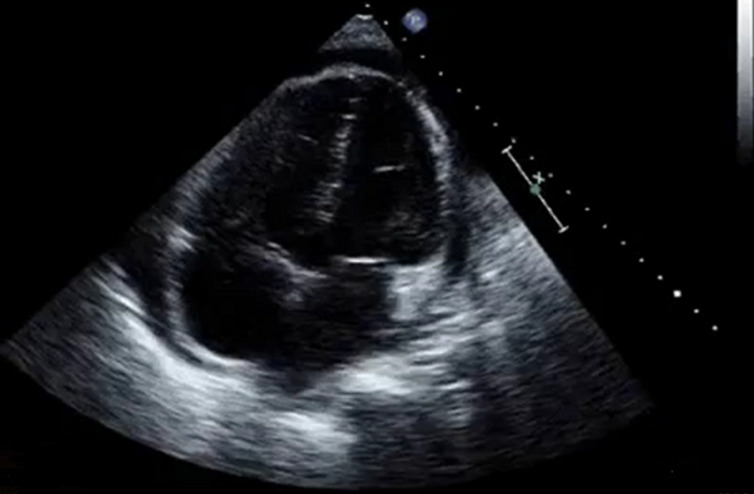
echocardiogram demonstrating circumferential pericardial effusion with thickening of the myocardium of the right ventricular wall
